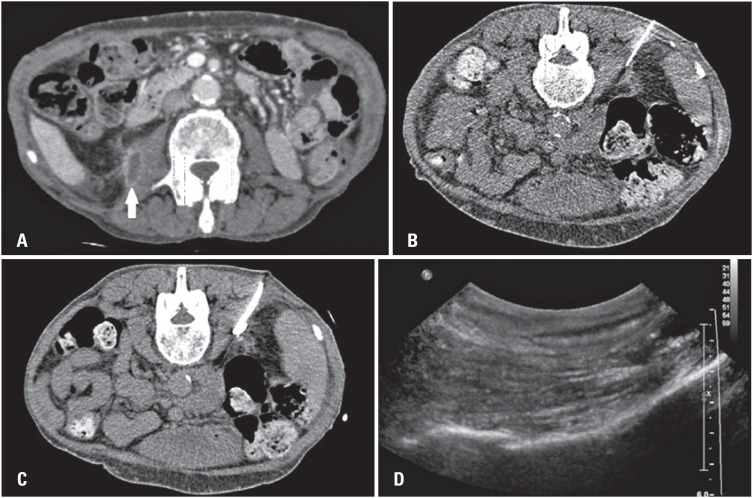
Percutaneous computed tomography-guided drainage using Seldinger technique. (A) Contrast-enhanced abdominal computed tomography showed a small fluid collection inside right psoas (arrow), (B) Final imaging control demonstrating well positioned Dawson-Mueller drain
